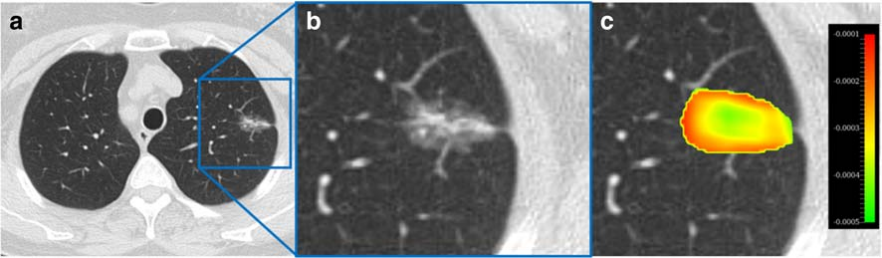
a CT axial view of a pulmonary nodule; b zoomed in view; c feature map overlaid on the zoomed in CT
